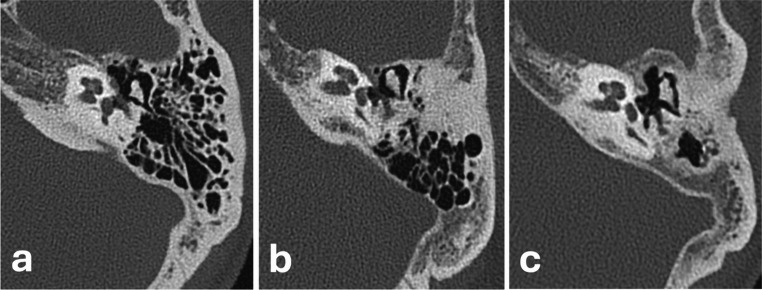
Mastoid pneumatisation. Axial CT showing well-pneumatised (a), moderately pneumatised (b) and poorly pneumatised (c) mastoid air cells, the latter of which may increase surgical difficulty during cortical mastoidectomy by limiting working space, hence increasing risk of facial nerve injury
